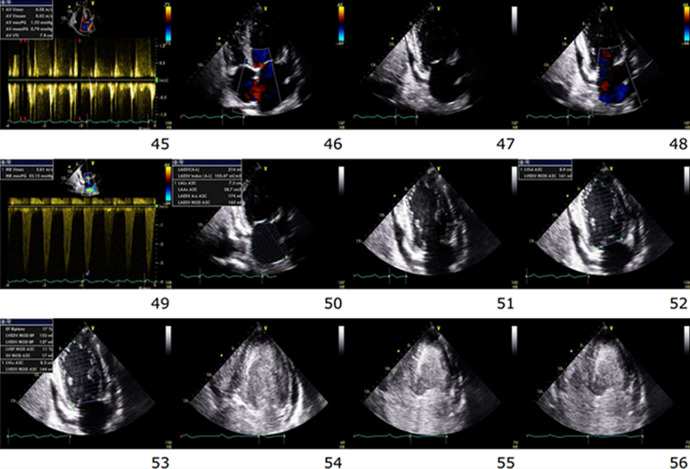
representative frames of echo images obtained during ACLS procedures; image 46 is an apical 4-chamber view showing a small amount of circumferential pericardial effusion present (47 - 48, 51 - 56); there was an abrupt appearance of SE, which begins in frame #54 in the LV, and persists until the final heartbeat
